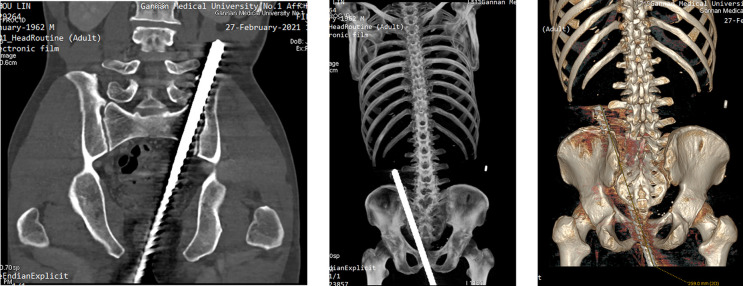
Abdominal CT scan + enhancement + post-processing (image reconstruction) showed that metal penetrating injury of left perineum, pelvis, left sacrum and ilium, the left internal iliac artery distal segment was not clearly displayed due to the artifact, and the relationship with the foreign matter was difficult to assess.
